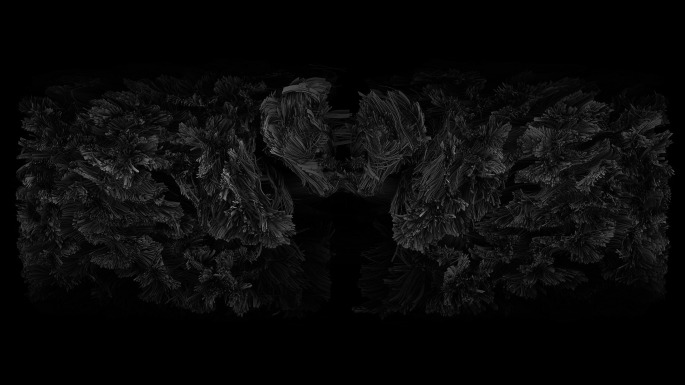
An MRI image from the Wellcome Collection of an unfolded adult brain. The image shows a Mercator transformation of the 3D space embedding a dense human brain tractography. The unfolding was performed from a ventral point of view and shows the arrival of white matter fibres to the neocortex. The cerebellum is at the top of the image at the midline. Immediately to the sides are the left and right temporal lobes, and the interhemispheric margin appears at the left and right borders of the image. Compiled by Katja Heuer and Roberto Toro
